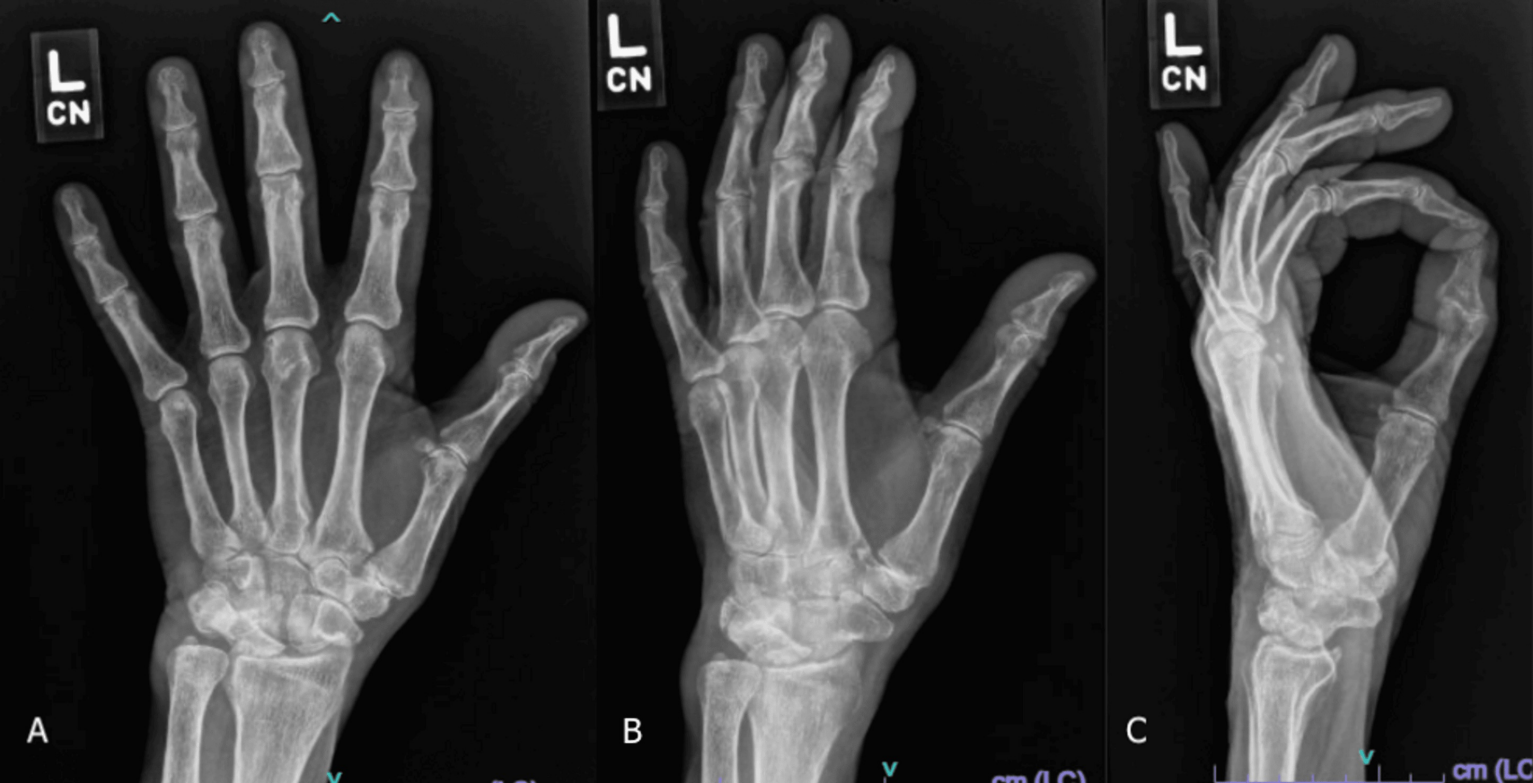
Preoperative left-hand radiographs. (A-C) Posteroanterior (PA), oblique, and lateral views demonstrate significant osteoarthritic changes of the first carpometacarpal (CMC) and radiocarpal joints.
